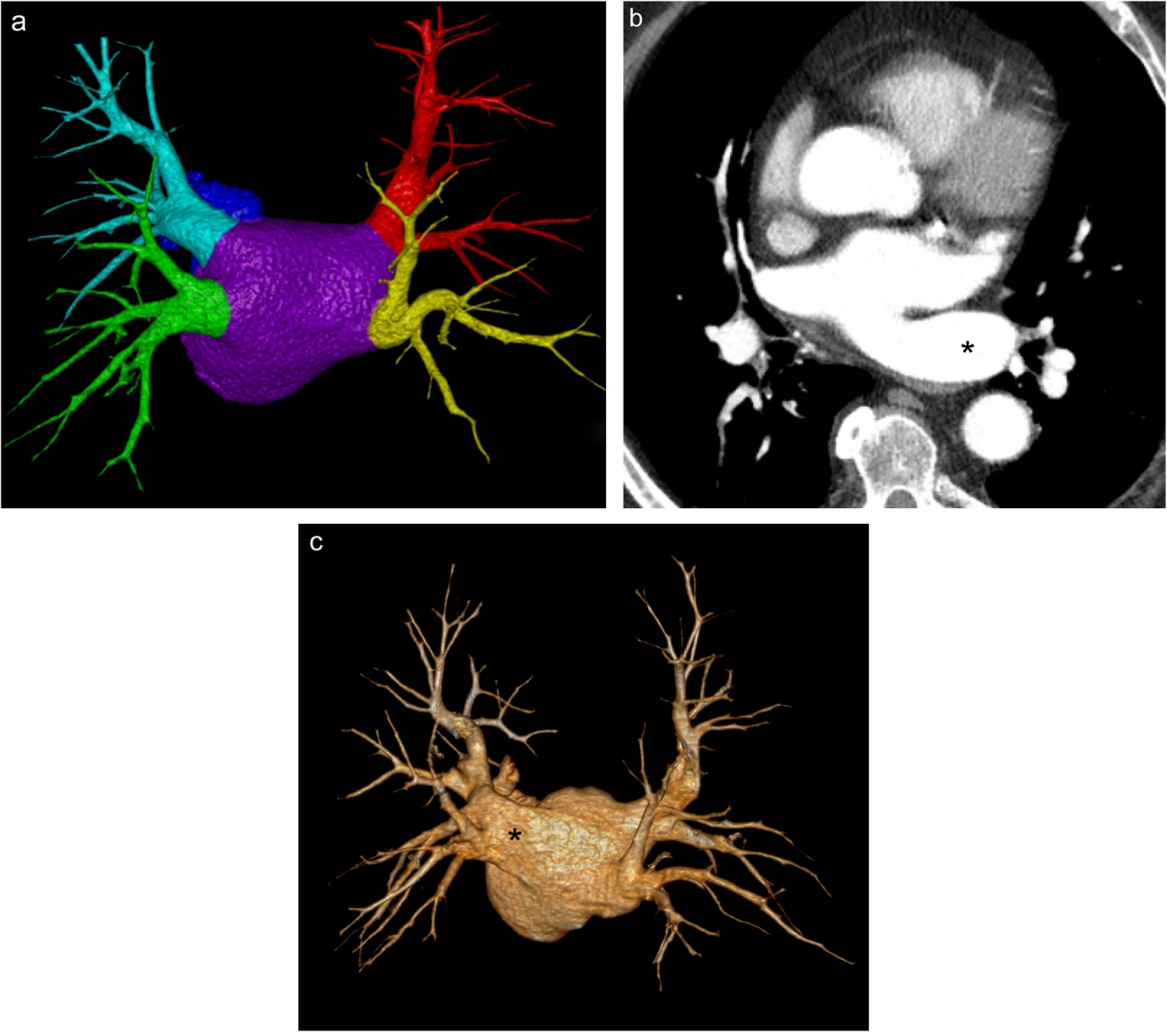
Pulmonary venous variant. a Three-dimensional reconstruction of a contrast-enhanced CT shows normal pulmonary venous anatomy with two right and two left pulmonary veins draining into the left atrium. b Axial CT image showing the most common variant of pulmonary venous anatomy, a fusion of the left pulmonary veins prior to entry in the left atrium (*). c Three-dimensional reconstruction shows a fusion of the left superior and inferior pulmonary veins with a shared ostium (*)
